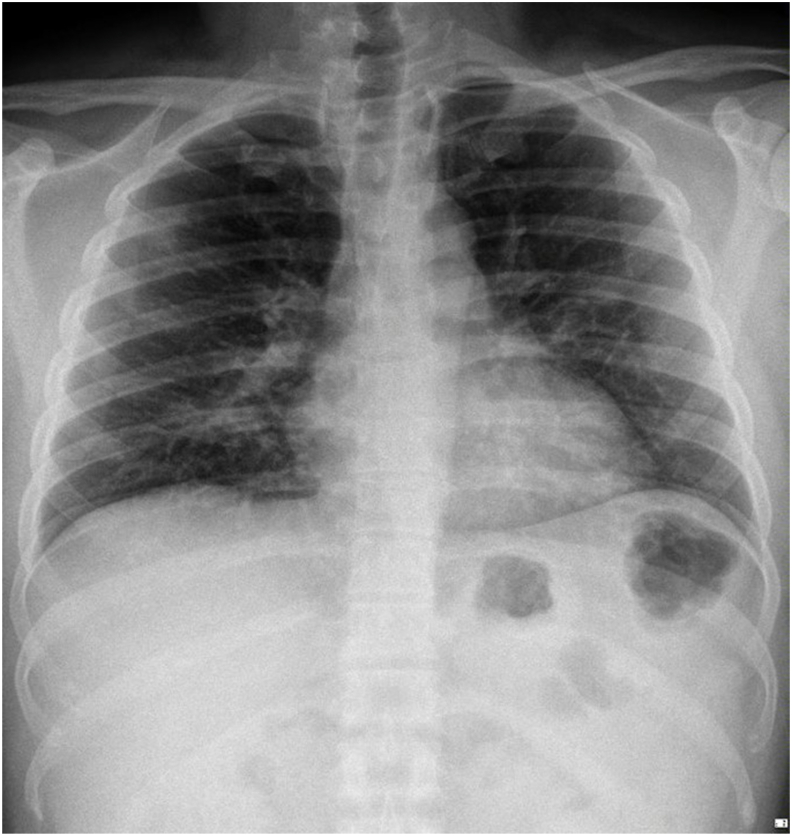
Chest X-ray indicated mild pneumonia in the right lung.
